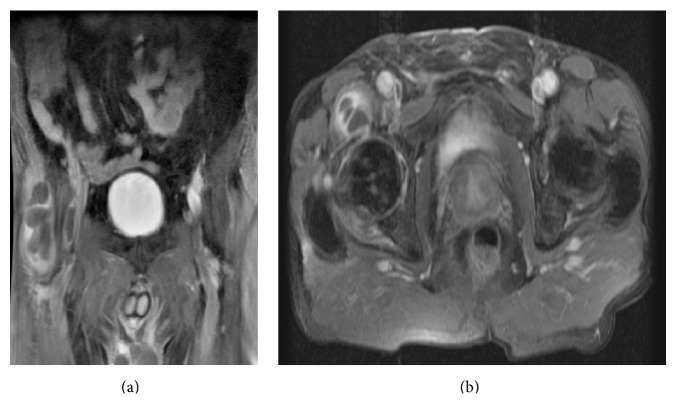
MRI with contrast. The MRI sequences with contrast medium confirm the predominantly fluid content of the neoformation, with contrast enhancement of the peripheral portion and the septa. Synovitis of the right hip is detectable. (a) Coronal postcontrast T1-weighted MR image with fat saturation. (b) Axial postcontrast T1-weighted MR image with fat saturation.
